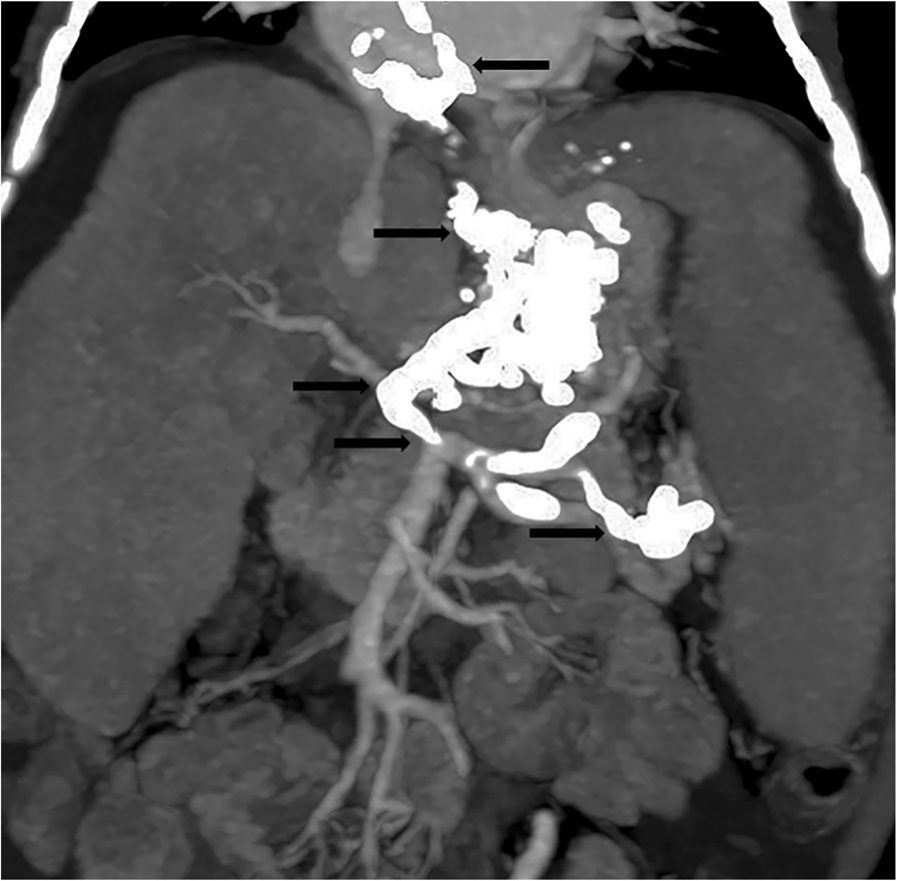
Abdominal computed tomography showing that the sclerosing agent was occluded in the esophageal varices, gastric varices, main portal vein, splenic mesenteric junction, and splenic veins (black arrows) and engorged the inferior mesenteric vein
